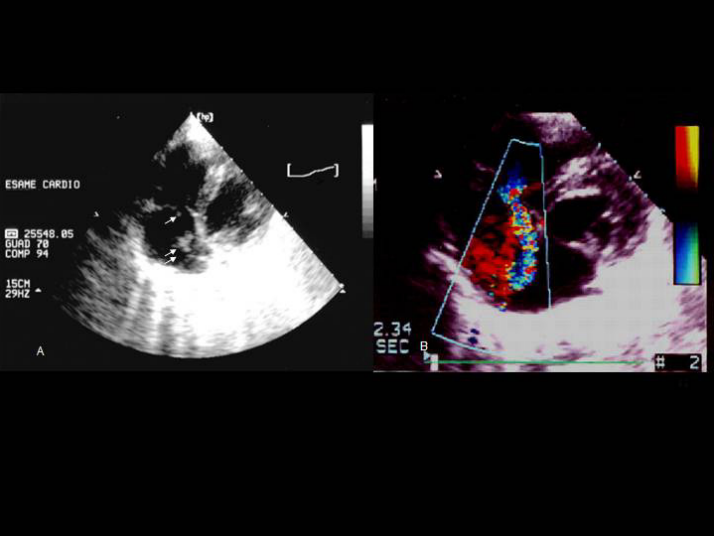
A, Two dimensional transthoracic echocardiogram (modified 4 chambers view) showing a vegetation (2.5 cm × 1.3 cm) on eustachian valve (double arrow) and another small vegetation (0.5 × 0.6 cm) on tricuspid valve (single arrow). B, Color flow doppler echocardiogram (modified 4 chambers view) showing a moderate tricuspid regurgitation with flow toward the eustachian valve.
